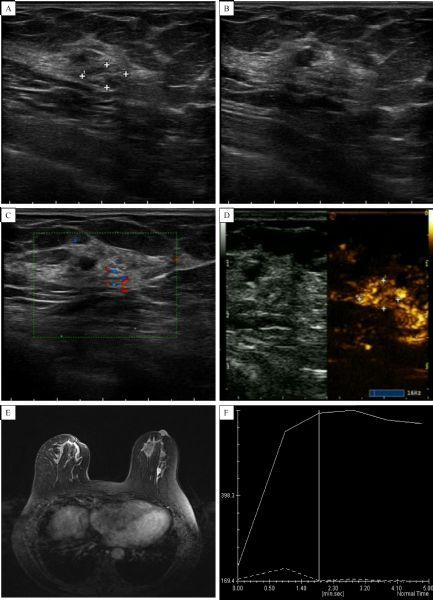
The feture of intraductal carcinoma. A-C: Conventional ultrasound showing multiple echogenic aggregation in the range of 9.5 mm × 5.0 mm, and small amounts of blood flow. D: In the same patient, after contrast-enhanced ultrasound, inhomogeneous and high enhancement was observed, with no significant enhancement surrounding the normal breast tissue. E: Enhanced MRI showing inhomogeneous and high enhancement; the curve exhibited a plateau. Pathology data showing intraductal carcinoma of grade Ⅱ-Ⅲ with two small infiltrates, and an infiltrating area of < 1 mm.
